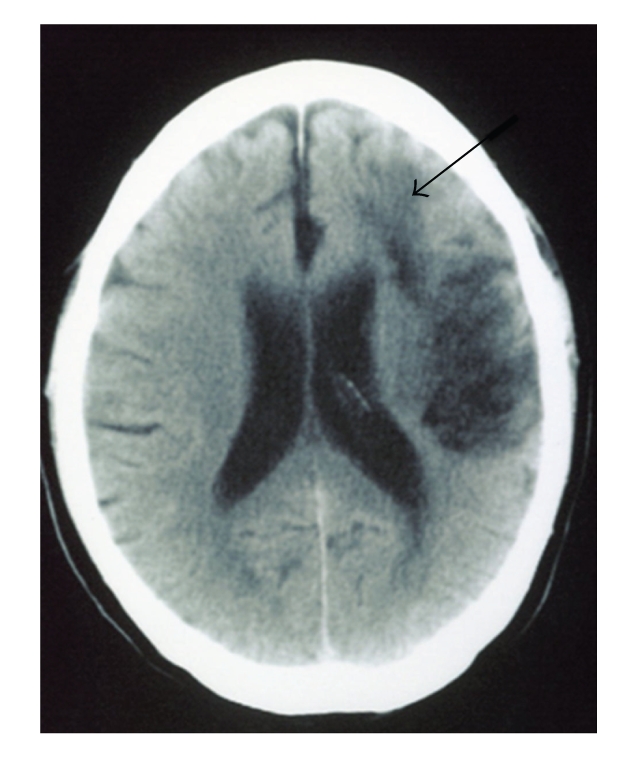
Axial Computed tomography of the brain 13 days after the carotid endarterectomy, demonstrated a new infarct in the white matter of the left frontal lobe (arrow).
